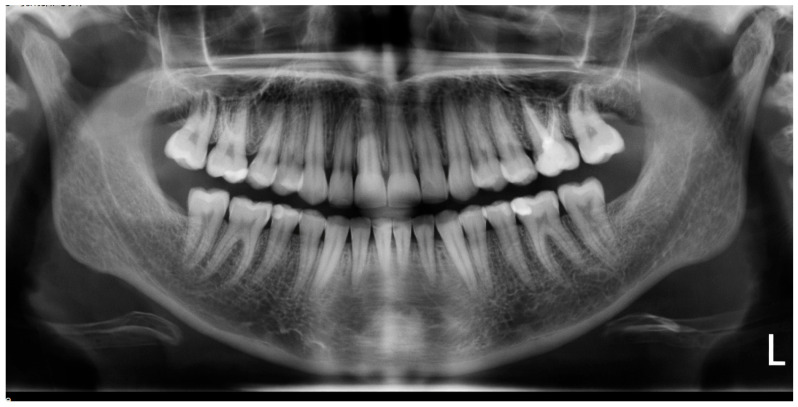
Representative digital panoramic radiograph obtained under standardized positioning conditions (Carestream Dental 8100). A root canal treated tooth is visible.
